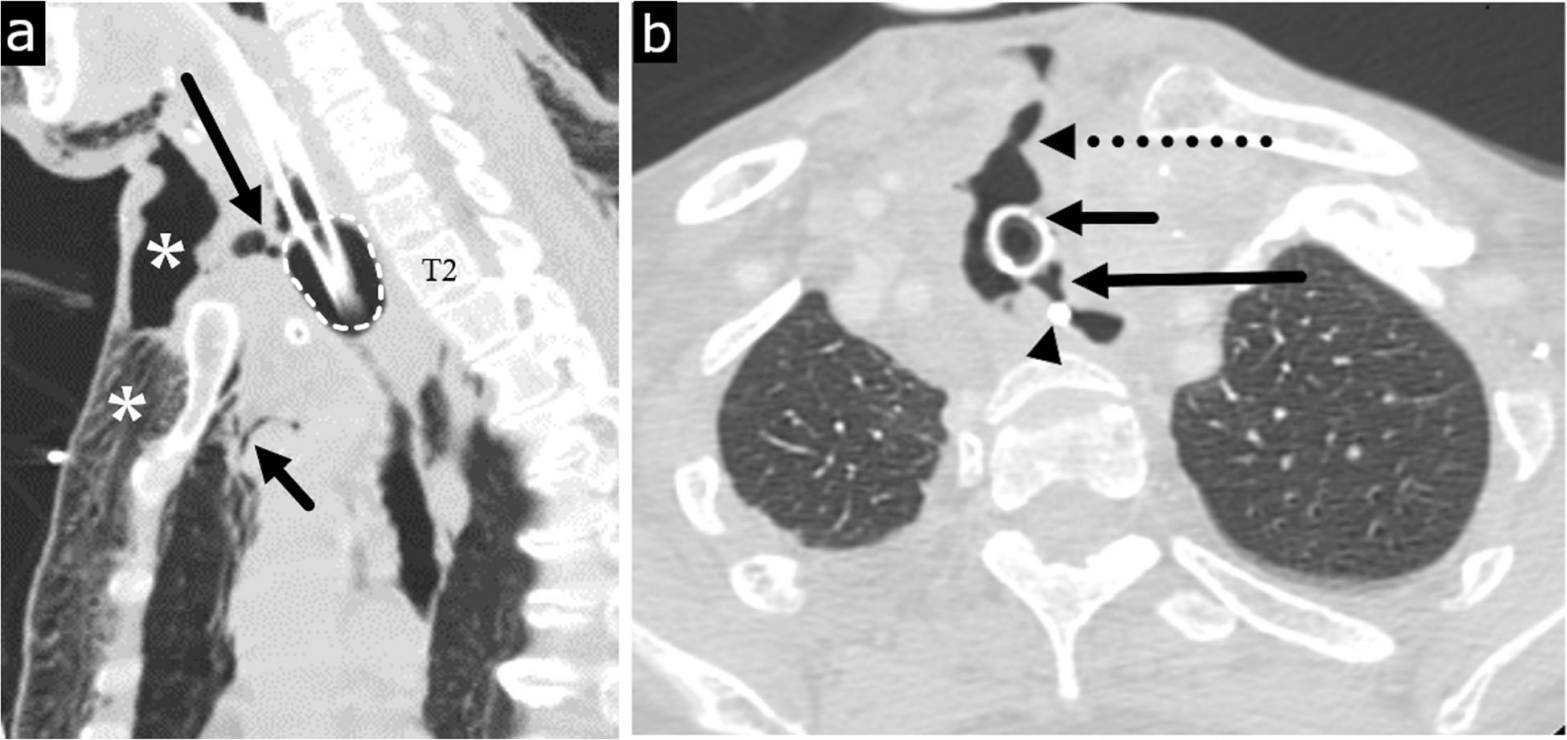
Tracheoesophageal fistula. Ninety-one-year-old female with long term intubation with subcutaneous crepitus. a Sagittal CT image shows a traumatic anterior tracheal wall defect (long black arrow) caused by an overinflated endotracheal tube balloon (dotted circle) resulting in a tracheocutaneous fistula and severe anterior chest wall subcutaneous emphysema (asterisks). Small pneumomediastinum (short black arrow) is seen. b Axial CT image obtained 3 weeks later shows a new tracheoesophageal fistula in the region of the previous overinflated endotracheal tube balloon (long black arrow), and a persistent tracheocutaneous fistula (dashed black arrow). Tracheostomy tube (short black arrow). Enteric tube (arrowhead)
